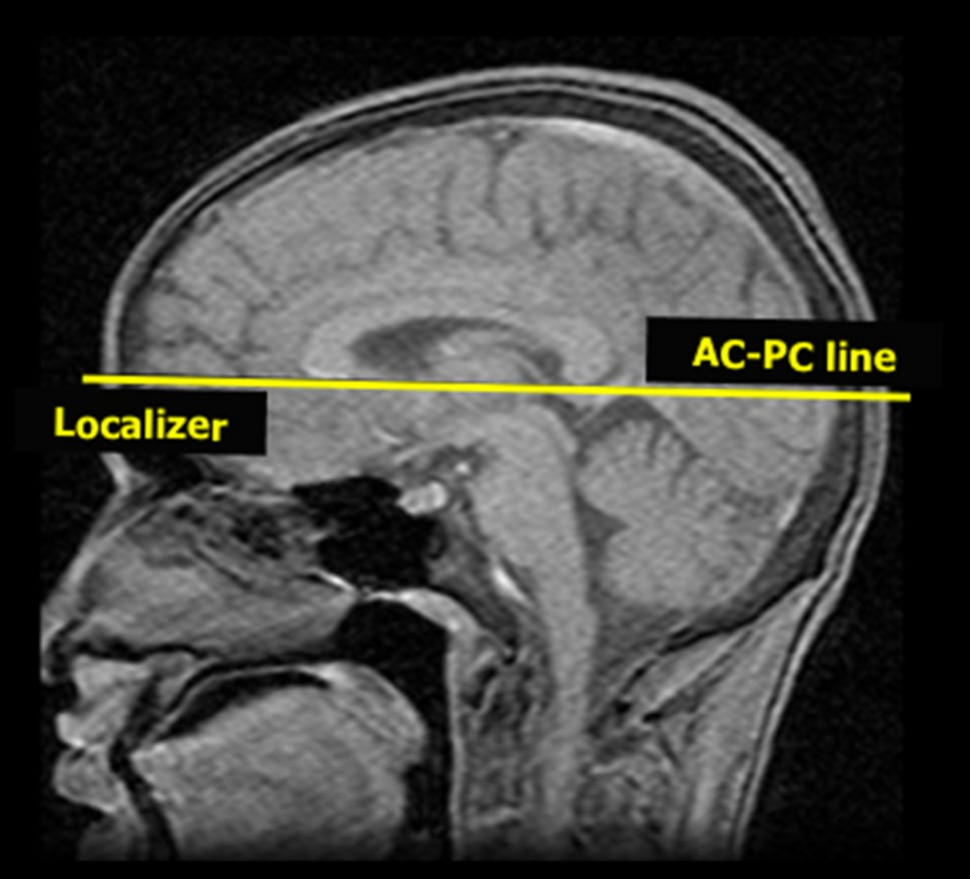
Head position during MRI acquisition. The anterior commissure–posterior commissure (AC–PC) line should be used to align head position to allow scan comparison, when only 2D acquisitions are possible. This line is typically ~ 9° steeper than the orbito-meatal line, traditionally used in computerised tomography (CT) scans. *MRI* magnetic resonance imaging, *2D* 2 dimensional
